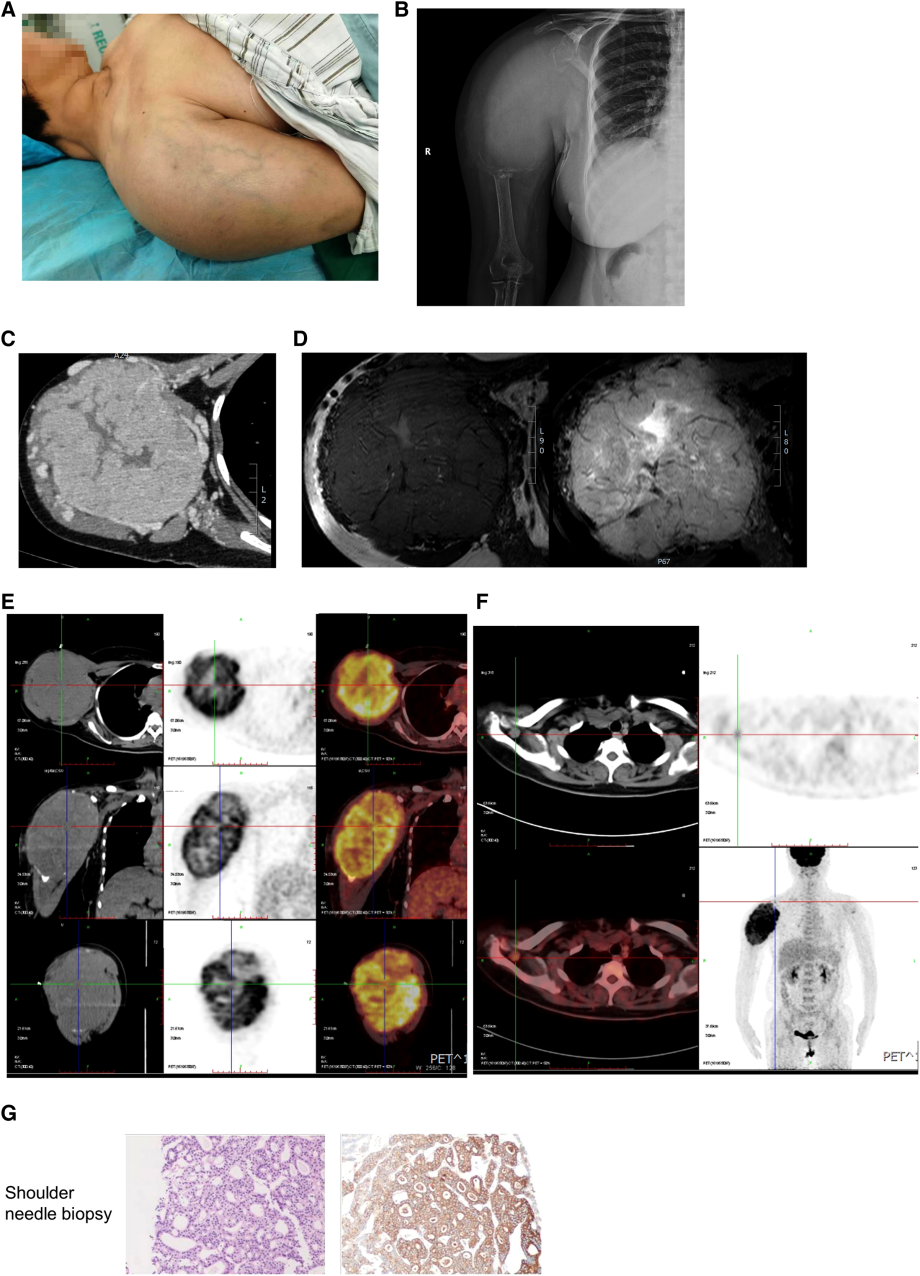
Examination and pathology of shoulder tumor. (A) The appearance of right shoulder. (B) The x-ray of the right humerus showed a vague high-density shadow with unclear boundary, and bone destruction near humerus and scapula. (C) Contrast-enhanced CT of the right humerus showed a soft mass replacing the normal bone structure with unclear boundary and dilated blood vessels. (D) No normal structure was found in the right humerus, shoulder joint and clavicle by MRI. T1 weighted image shows a mass with low signal intensity. T2 weighted image shows high signal intensity. (E,F) ^18^F-FDG PET/CT image showed that increased uptake in shoulder tumor (E) and the right supraclavicular fossa (F). (G) Needle biopsy of shoulder mass.
